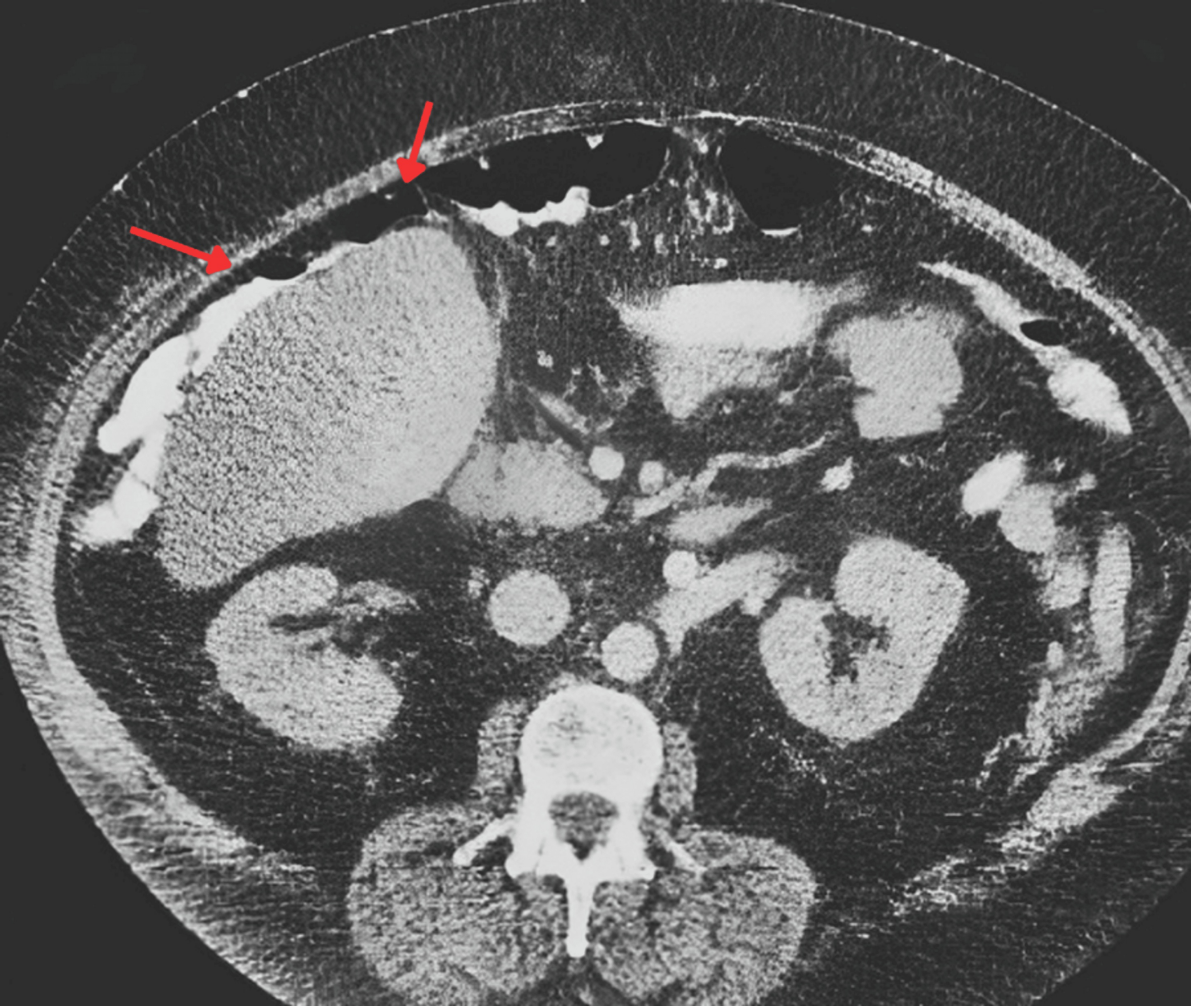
Computed tomography (CT) displaying the left colon interposition between the liver and the left diaphragm (indicated by the arrows).
